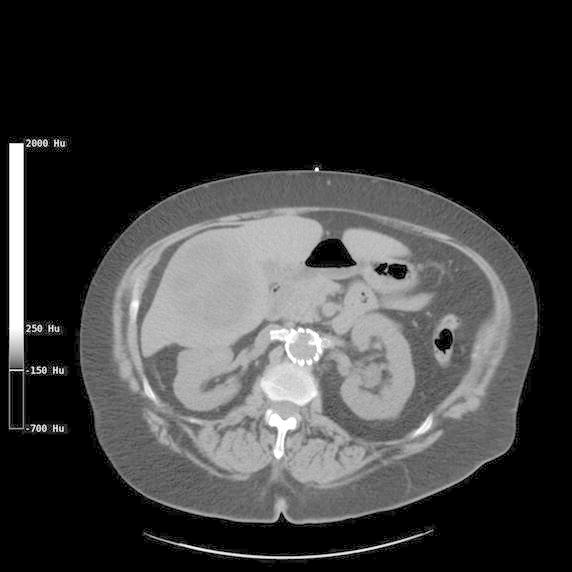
Abdominal computerized tomography (CT) showing a multiseptated thick walled mass in the right hepatic lobe.
